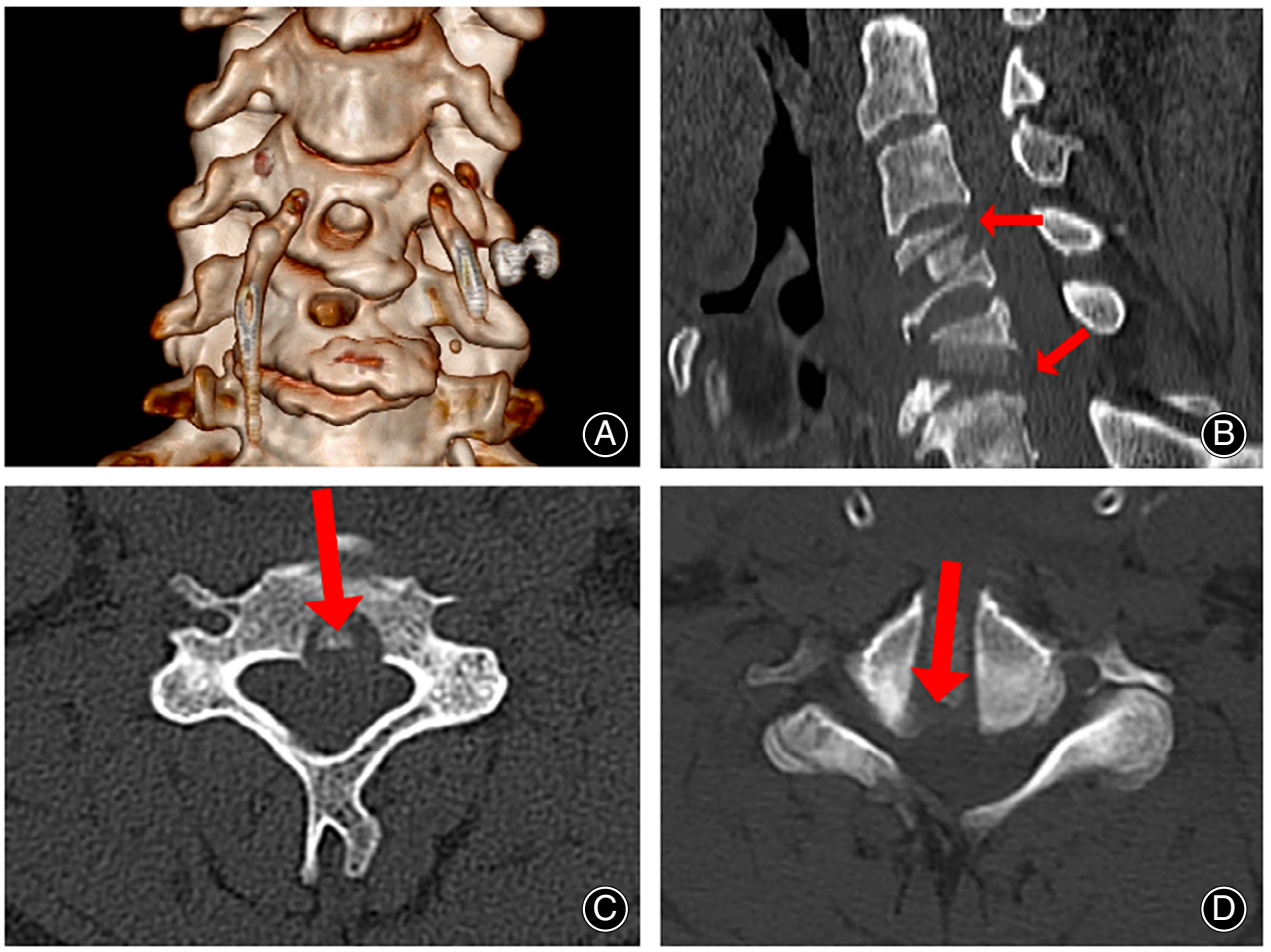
Postoperative CT image. Postoperative CT three‐dimensional reconstruction shows the entry location of the bone channel location (A). The sagittal position showed 3/4 and 5/6 cervical intervertebral disc‐osteophyte complex removed, showing the location and orientation of the channels (arrow) (B).The transverse view showed that the C4 and C5 vertebral bodies were complete in the channel, and the bone graft was implanted (arrow) (C, D).
